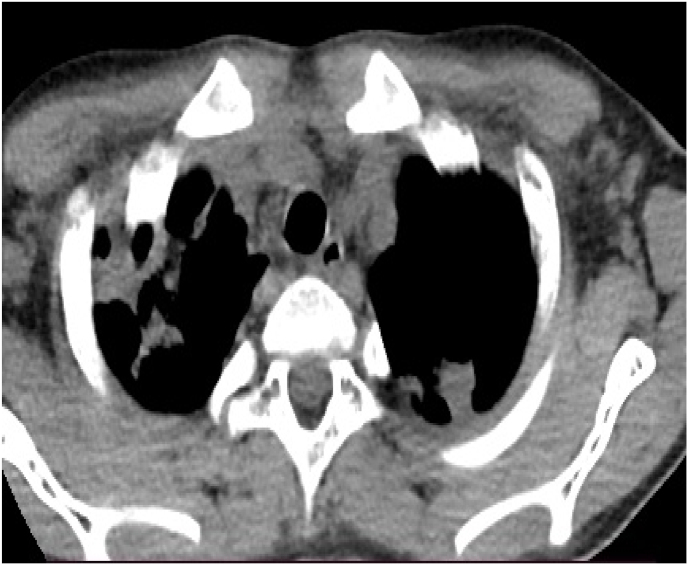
Axial CT of the chest (mediastinal window) showing bilateral pulmonary cavities in the upper lobes surrounded by circumferential pleural thickening.
